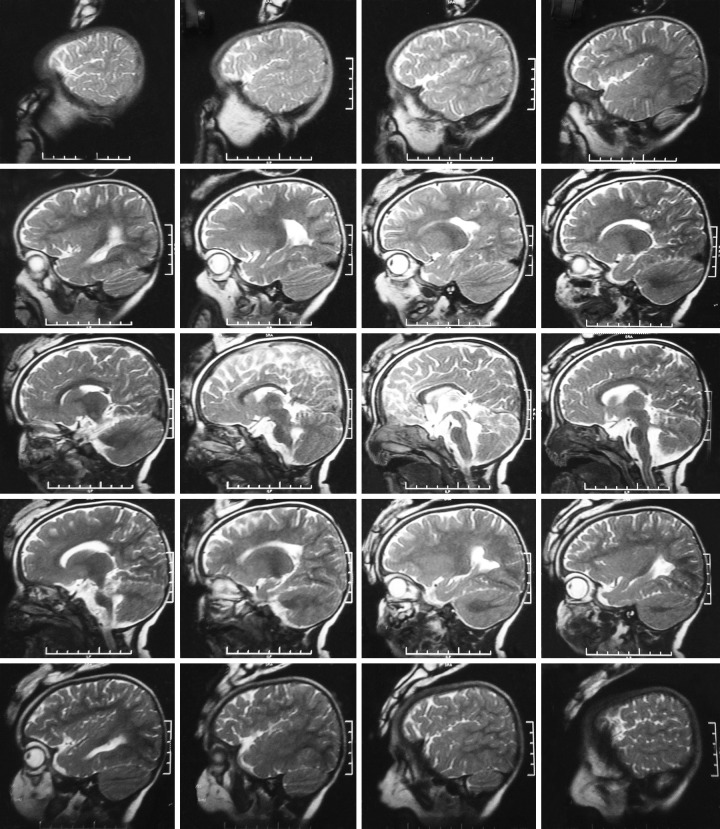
Computed tomography scan of brain
